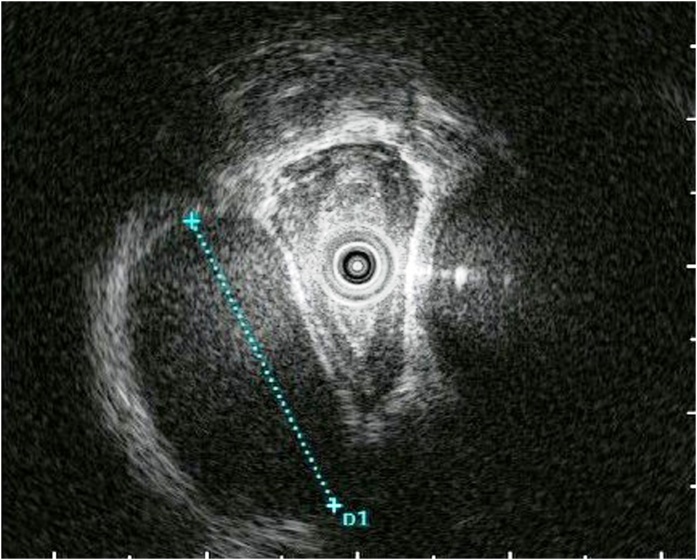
Endoscopic ultrasound findings. EUS image showing a cystic tumor in the esophageal mucosa, with no abnormality, nodules, or partitions within the cyst (dotted line).
